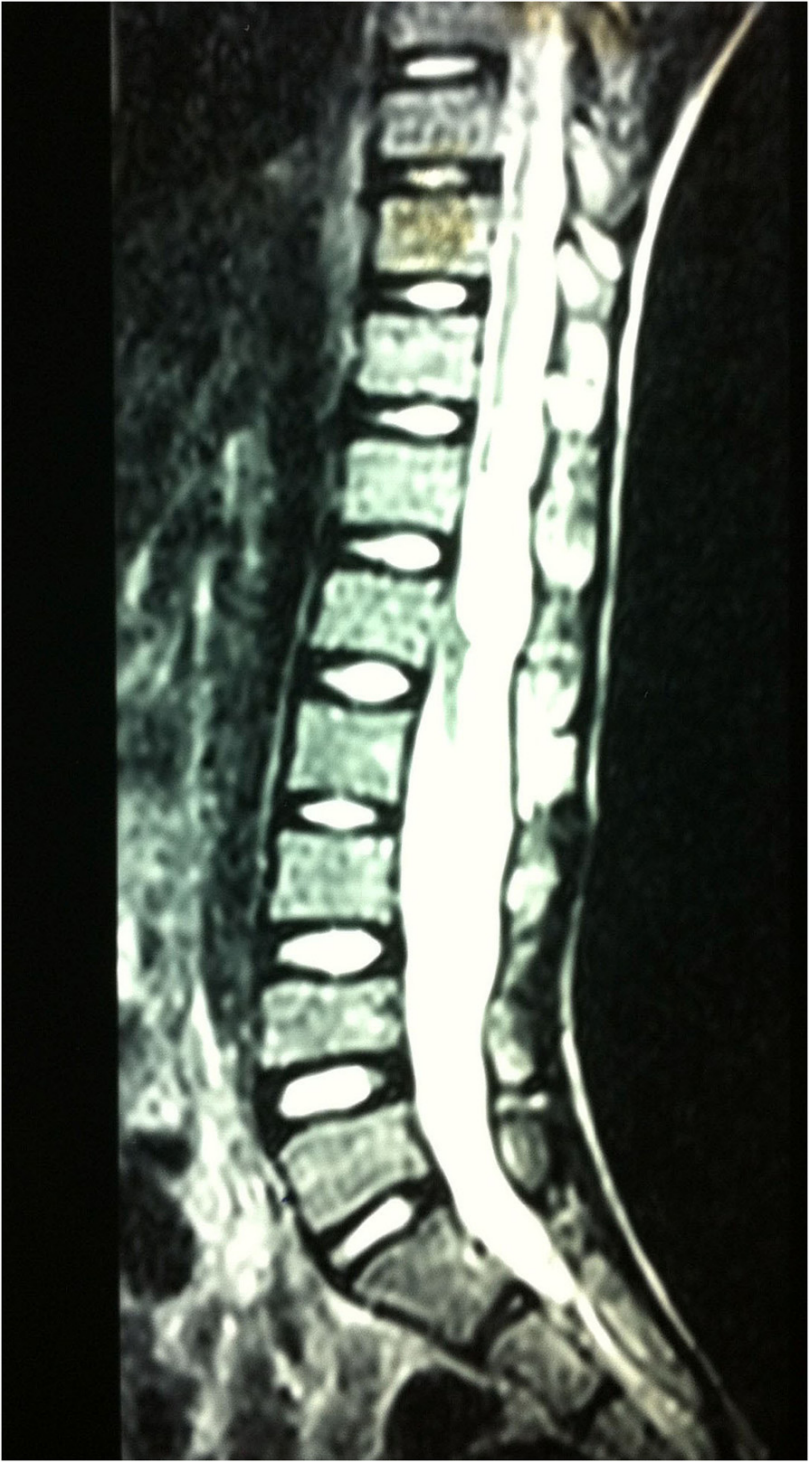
Sagittal T2-weighted axial image showing a homogenously hyperintense lesion.
